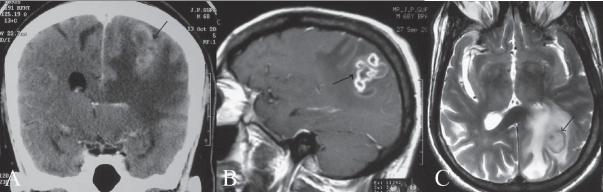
Coronal contrast-enhanced CT (A), contrast-enhanced sagittal MRI (B), and T2W axial MRI (C) images of the brain show conglomerate ring-enhancing lesions in the left parietal lobe, with significant surrounding edema and contralateral shift (arrows in A and B). Note the central hyperintense signal with low signal intensity walls (arrow in C)
